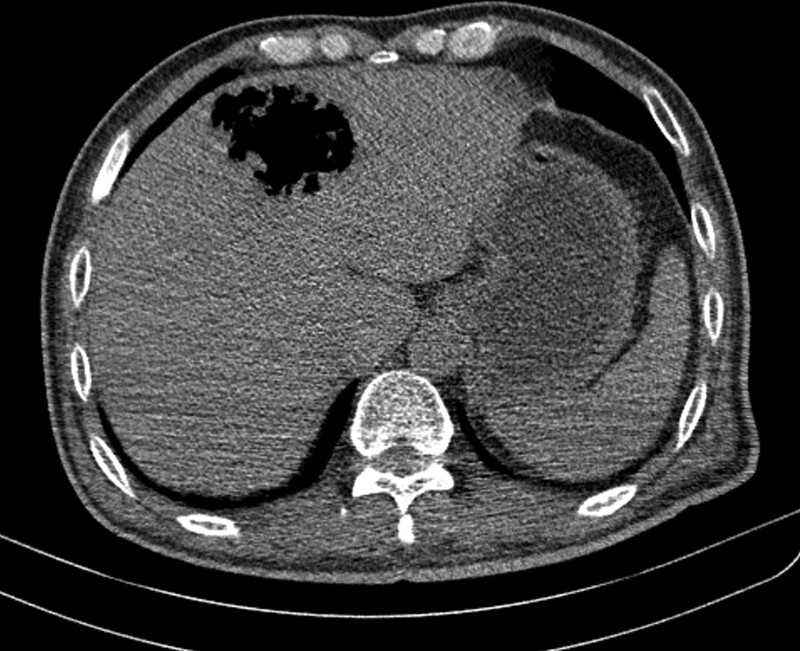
Initial computed tomography showed a large scale of air collection in the left liver lobe without fluid.
